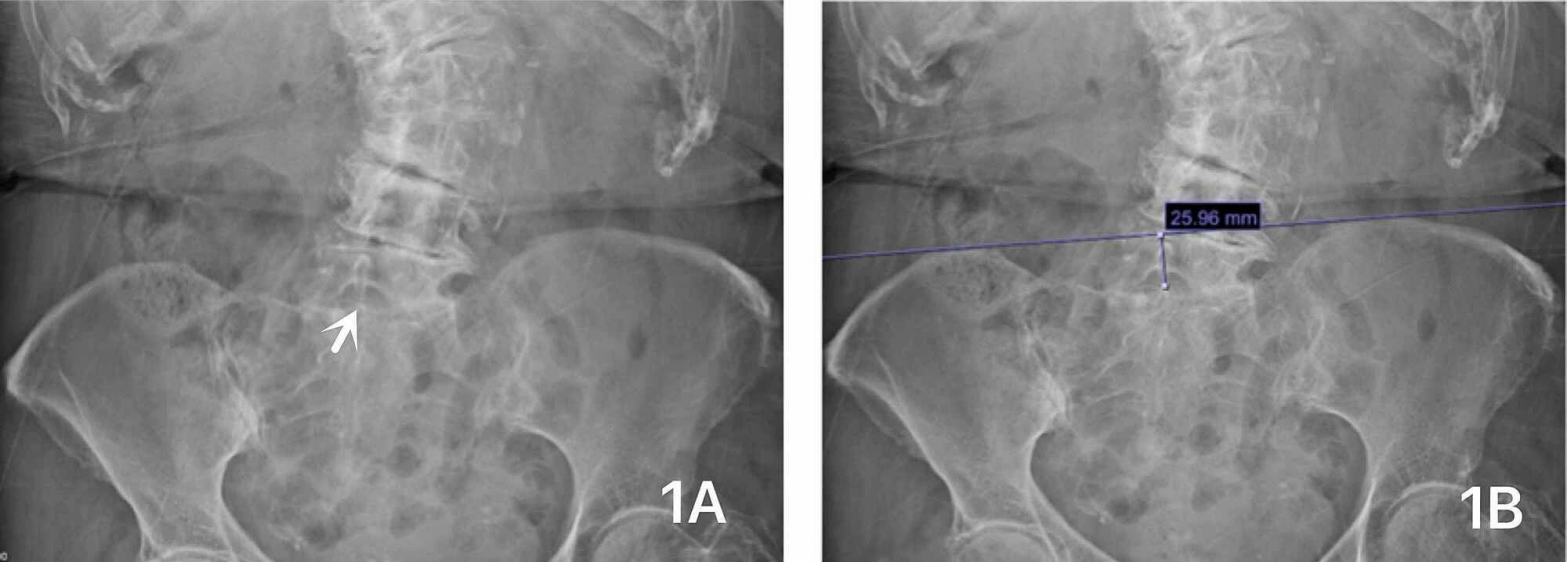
Patient’s lumbar x-ray. Figure 1A shows large radiographically patent L5-S1 interspace (arrow) with notable severe lumbar scoliosis, and Figure 1B shows the measurement of the distance between iliac crests and L5-S1 interspace.
